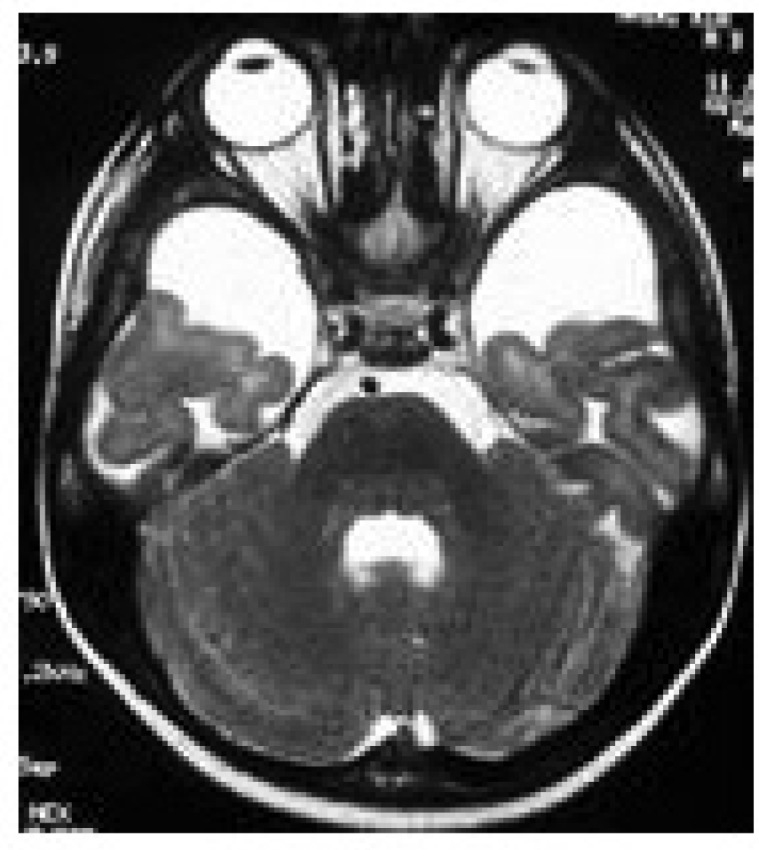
Chronal T2 weighted MRI in patient 7 shows bitemporal arachnoid cysts
